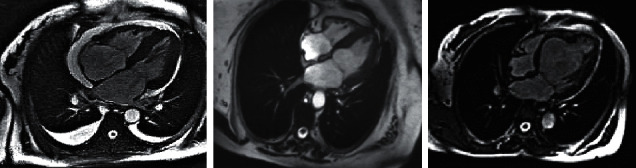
MRI images of patients with myocardial injury.
